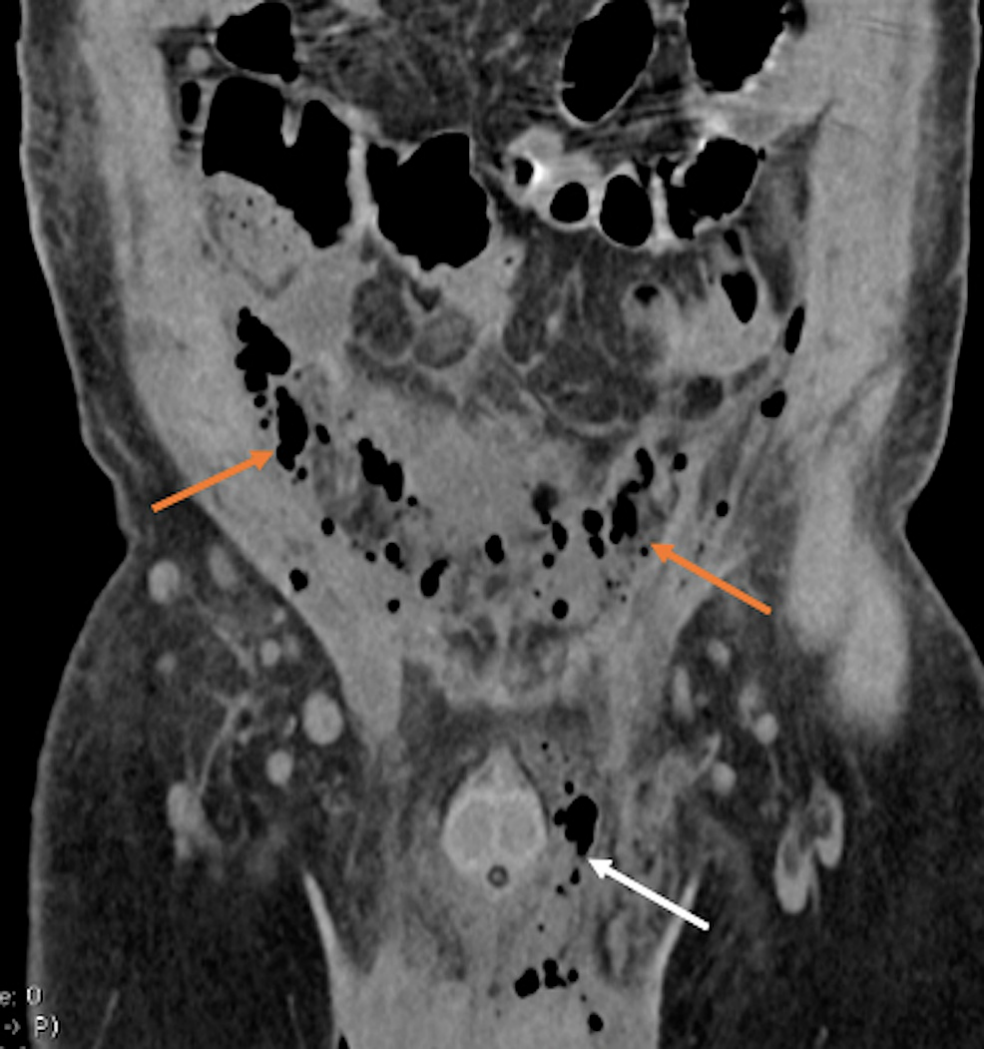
Non-contrast abdominal CT in coronal view. Multiple emphysema foci spreading from the genital area (white arrow) to the peritoneum (orange arrows). These findings are consistent with an intraperitoneal extension of FG. CT: computed tomography; FG: Fournier’s gangrene
